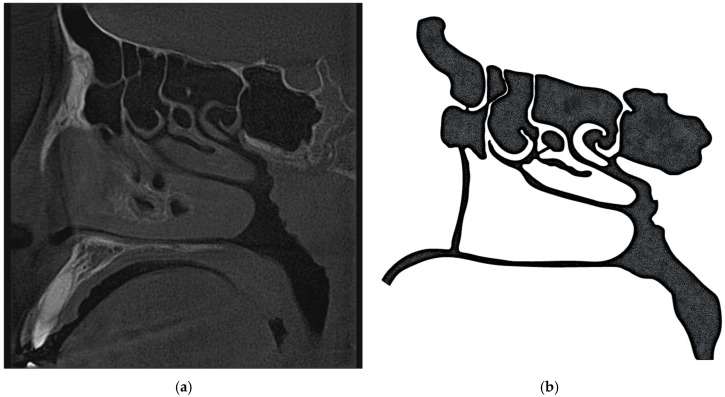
(a) CT-scan images of a patient; (b) CFD domain generated based on the CT-scan image.
